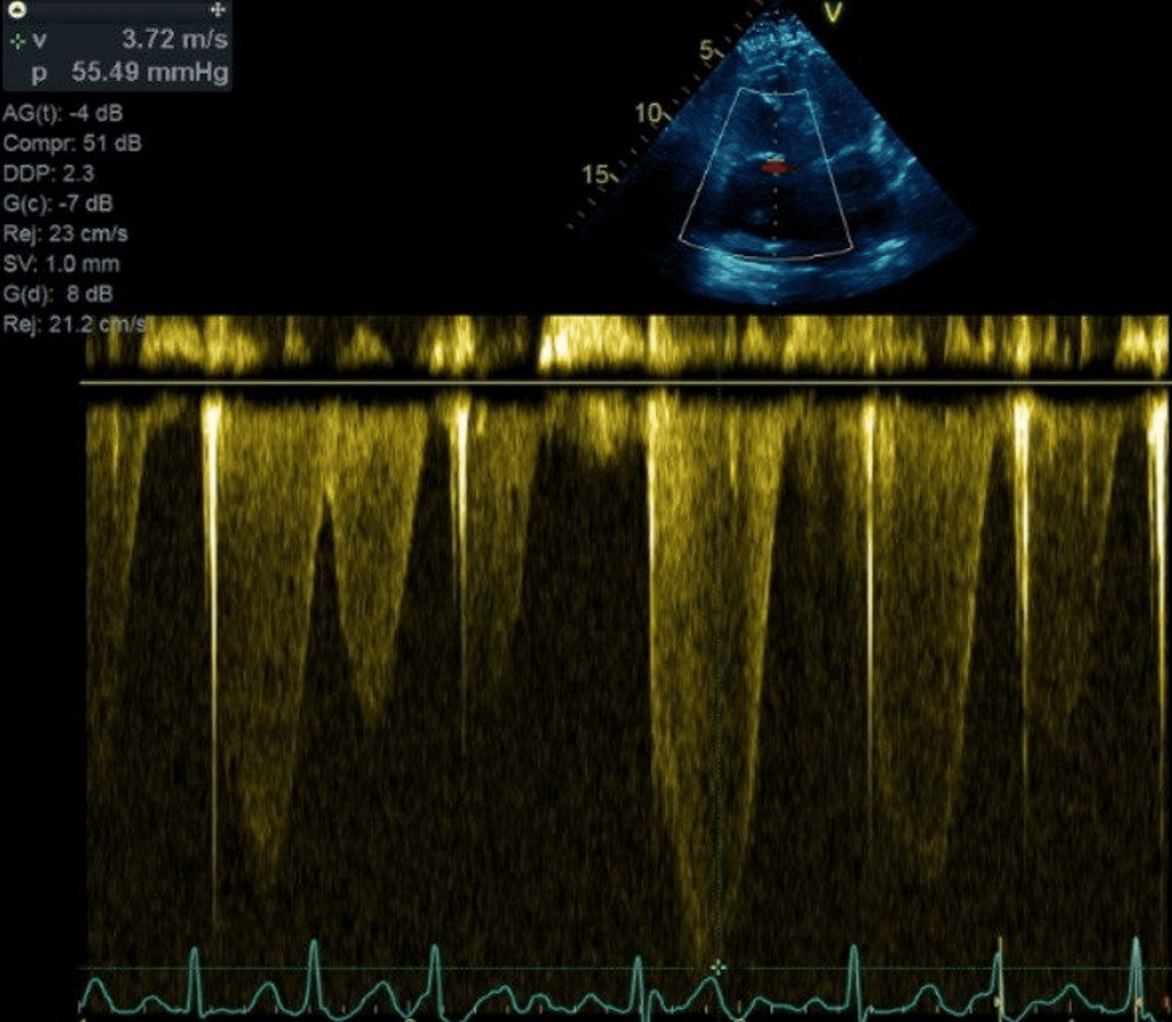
Doppler across the tricuspid valve showing TR with velocity of 3.7 m/sec, indicating an elevated pulmonary pressure. TR: tricuspid regurgitation
